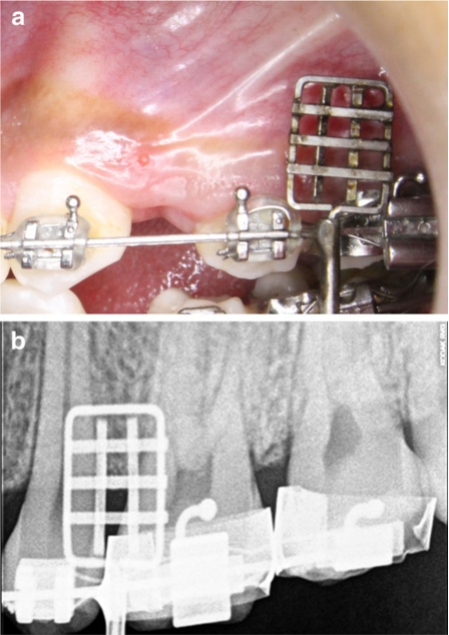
Custom made guide in second premolar and the first molar region. As seen (a) clinically and (b) radiographically.
